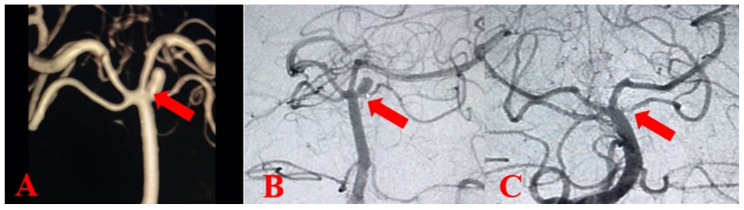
Intracranial aneurysm brain-computed tomography image (A), digital subtraction angiography image of intracranial aneurysm (B), intracranial aneurysm after endovascular coil procedure (C).
